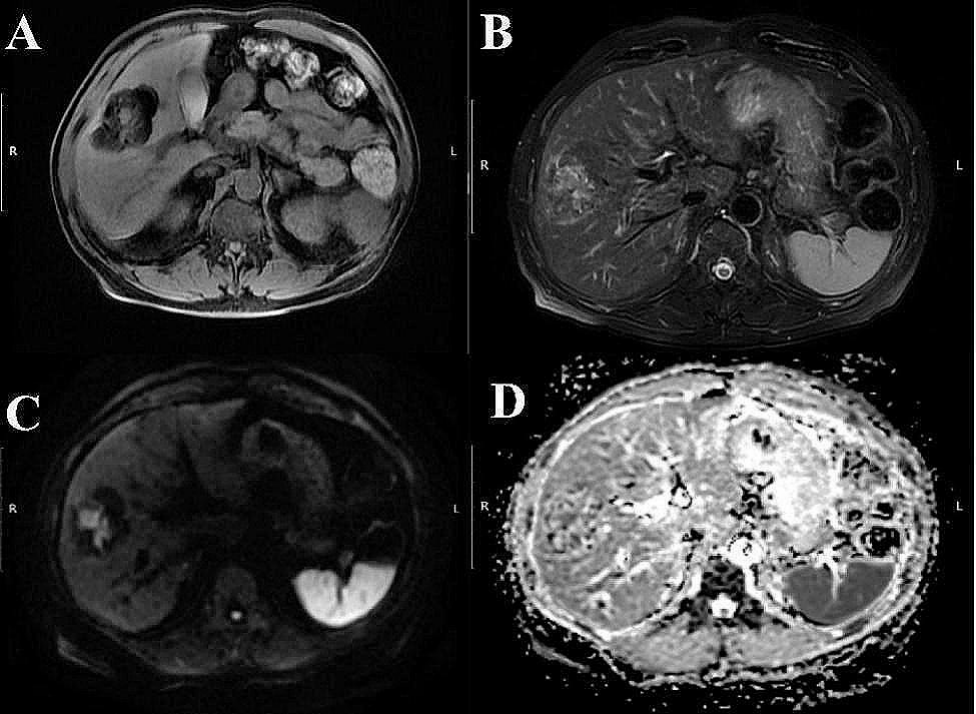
The MRI image depicts a benign hepatic PEComa, characterized by a round abnormal signal shadow measuring approximately 5.2 cm×6.7 cm×4.7 cm in the right lobe of the liver. This shadow appears lobulated and well defined, exhibiting predominantly mature fatty signal with interspersed masses of soft tissue signal. The image panels A, B, C, and D represent T1WI, T2WI, DWI, and ADC images, respectively
